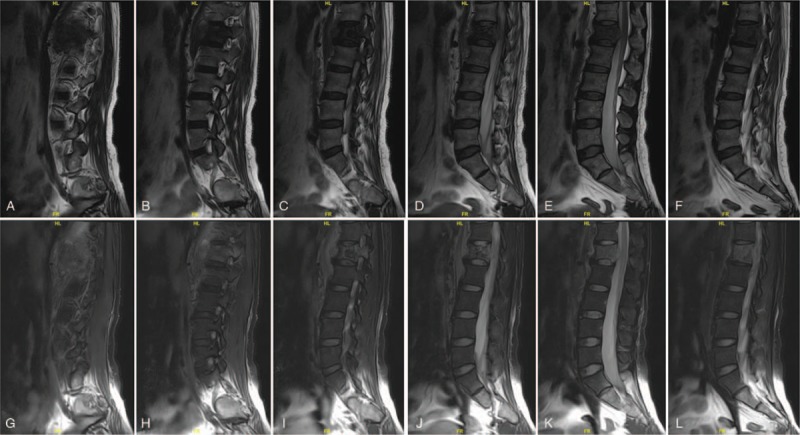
(A–L) Preoperative sagittal magnetic resonance imaging scan revealing the density of soft tissue, obvious bony destruction in the L1, and spinal cord compression caused by metastatic adrenocortical carcinoma, with increased metastatic marrow infiltration of the vertebrae and paravertebral regions.
